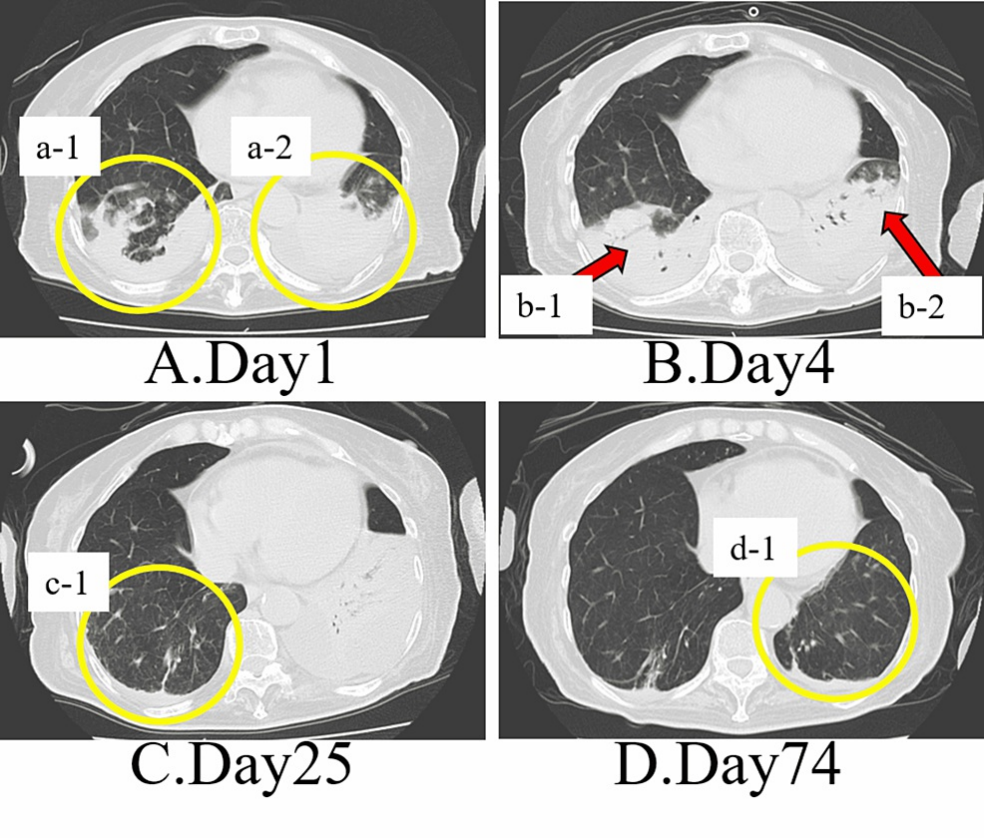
Chest CT on hospital admission day 1 (A), day 4 (B), day 25 (C), and day 74 (D). A. Bilateral pleural effusions, a small amount of pericardial effusion, and atelectasis in the bilateral lower lobes. Pneumonia is seen in the bilateral lower lobes (yellow circle a-1, a-2). B. Mildly increased left pleural effusion. Infiltrating shadows in the bilateral lower lobes are enlarged in extent and show exacerbation of pneumonia (red arrow b-1, b-2). C. Infiltrating shadow, atelectasis, and pleural effusion in the right lower lobe improved (yellow circle c-1). D. Improvement of infiltrating shadow and atelectasis in the left lower lobe (yellow circle d-1).
